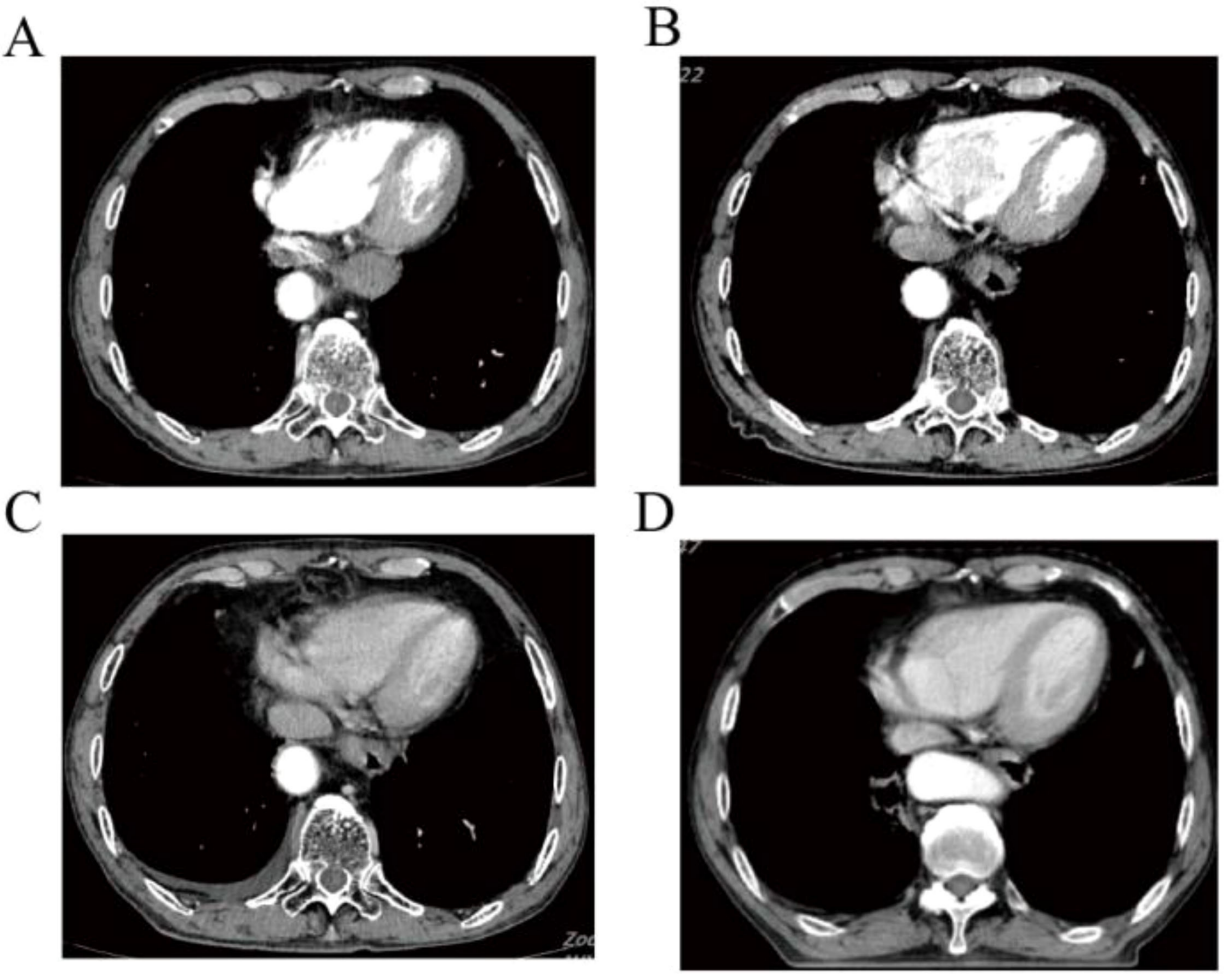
Chest CT of shows the changes of esophageal lesions of anti-tumor therapy. (A) Esophageal lesions before treatment. (B) Esophageal lesions after 2 cycles of chemotherapy combined with immunotherapy. (C) Esophageal lesions before radiotherapy. (D) Esophageal lesions on chest CT were reexamined after radiotherapy.
